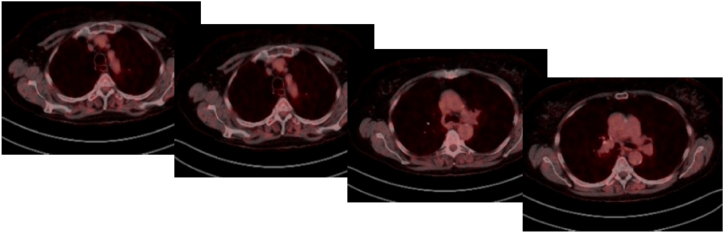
PET-CT-Scan with 1-year-follow up after resection of the right upper lobe: No detectable FDG avidity.
